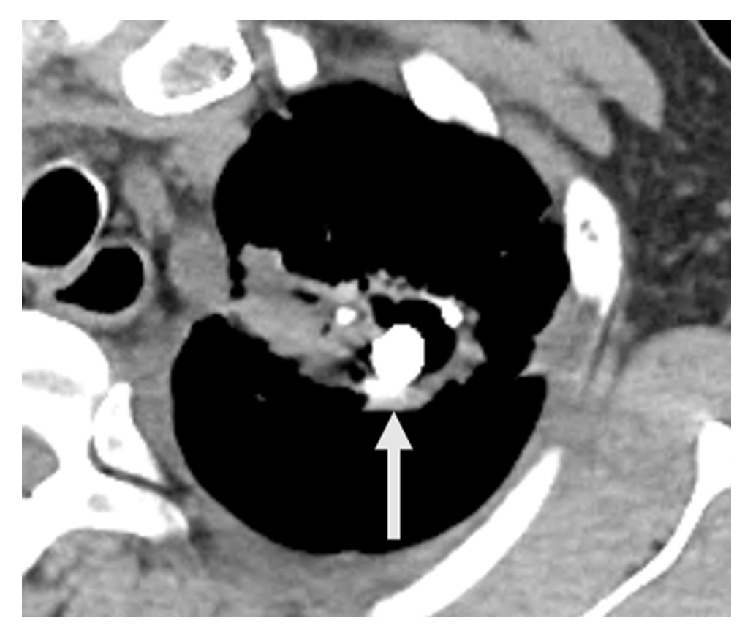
Non-contrast-enhanced axial computed tomography image. Two months after the treatment, the aneurysm is filled with the mixture of n-butyl-2-cyanoacrylate and iodized oil (arrow).
